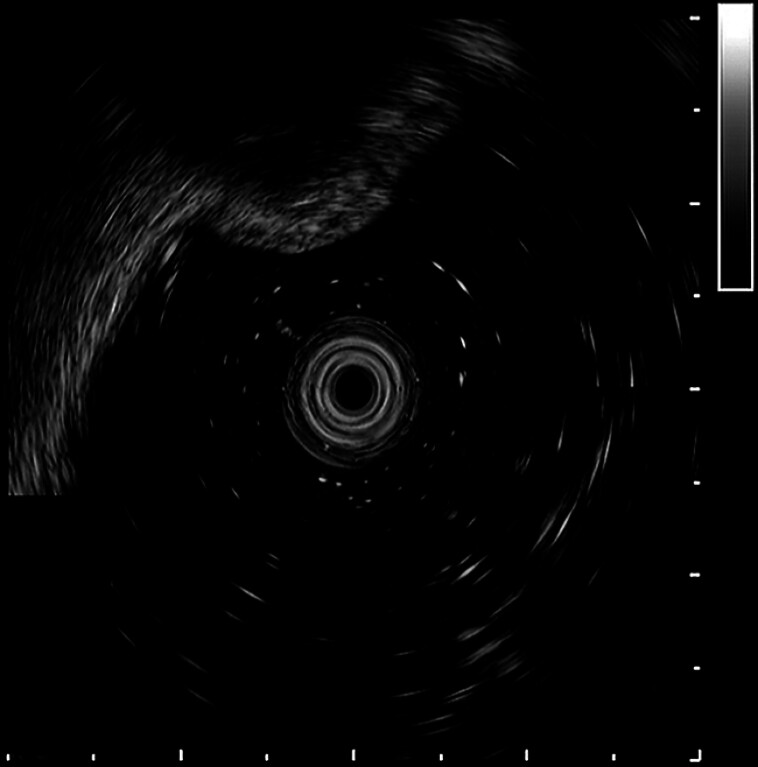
Ultrasonographic image showing the encapsulated fecalith between the submucosa and muscularis propria.
